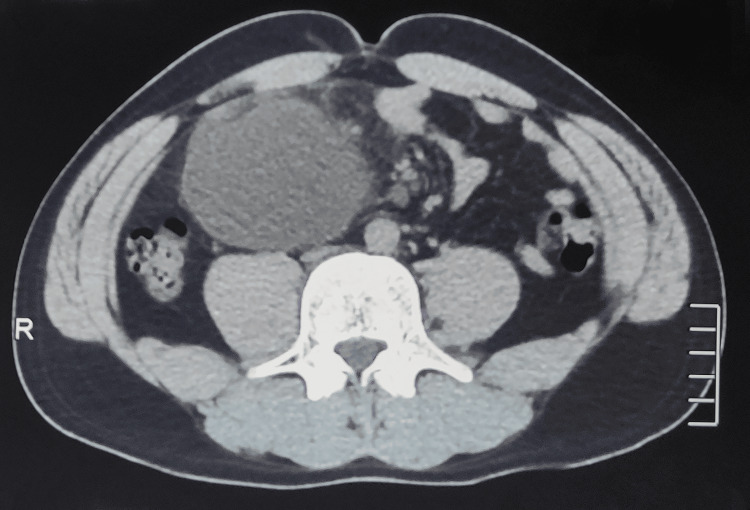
Abdominopelvic CT scan in the transverse section reveals a rounded cystic formation originating from the mesentery
